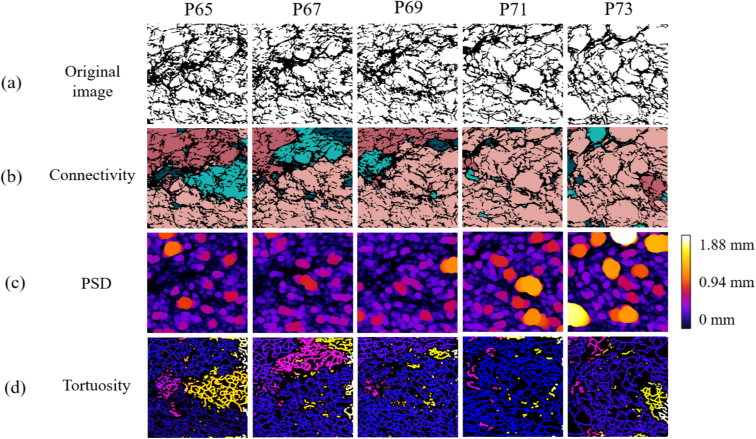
Structural analysis of bread CT scans.
